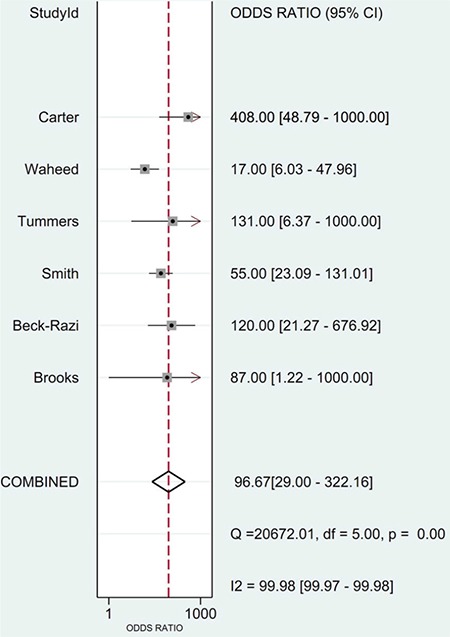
Summary of diagnostic odds ratio of focused assessment with sonography in trauma for assessing injury.
